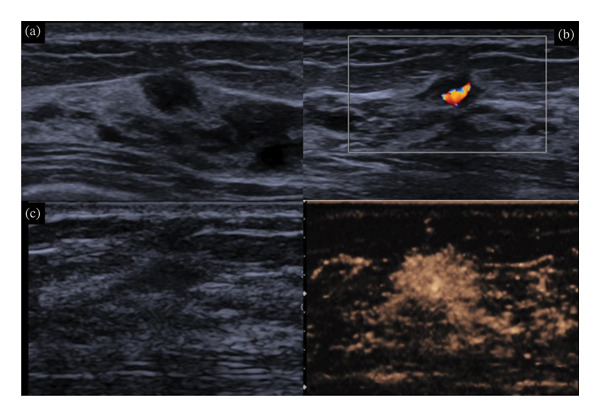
A 42‐year‐old lady with a BI‐RADS 4A lesion in the right breast. The maximal diameter of the lesion was only 6 mm. No discomfort was complained. In combination with contrast‐enhanced ultrasound, the lesion was upgraded to BI‐RADS 4B. Ultrasound‐guided core‐needle biopsy was performed. The pathology was intraductal carcinoma. (a) B‐mode ultrasound showed a hypoechoic lesion with microlobulation margin and (b) color Doppler detected vessels in the lesion, and (c) CEUS showed hyperenhancement with irregular shape and larger scope.
